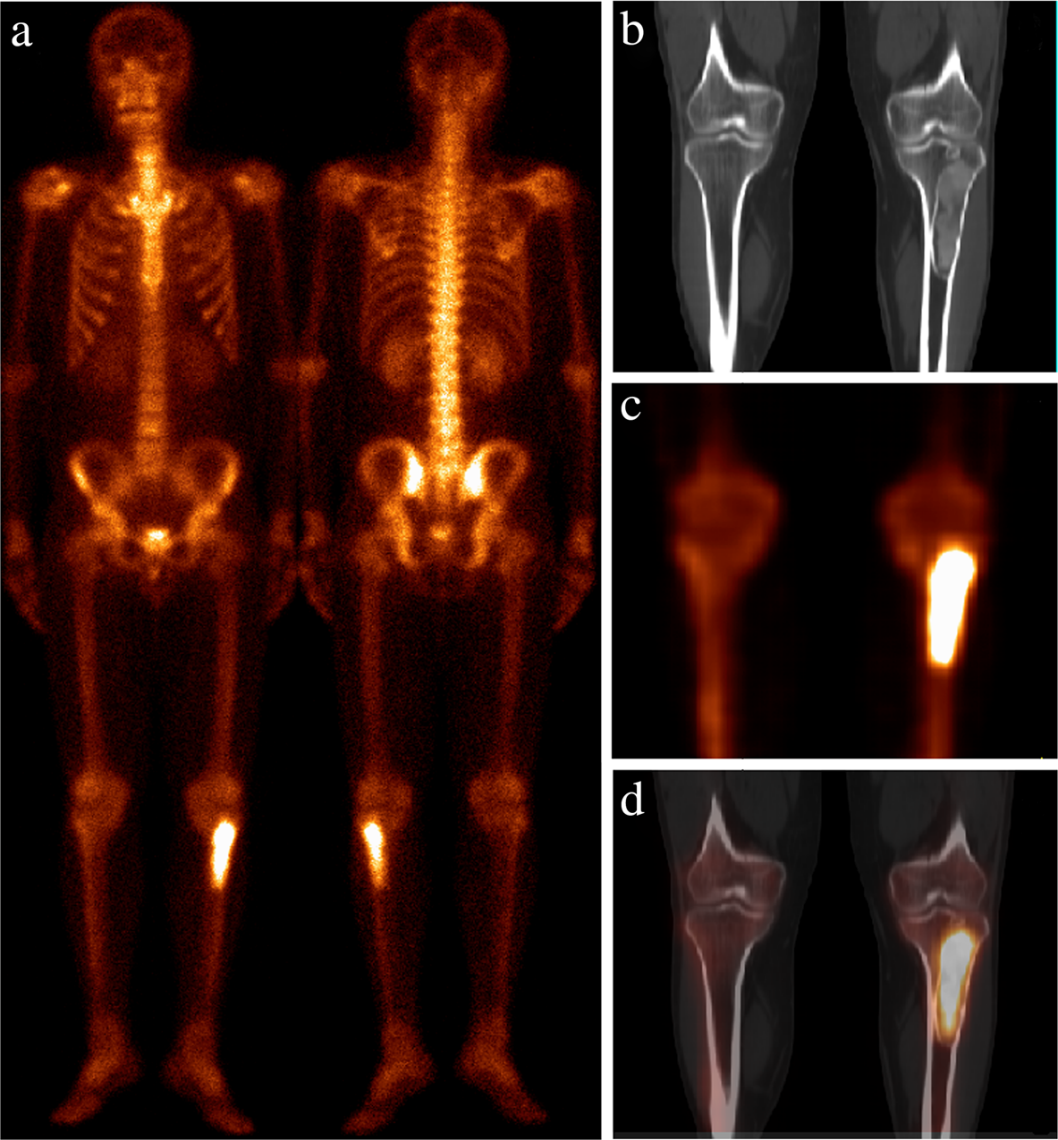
Patient 12 presented with NPC. On the WBS (a), there is an area of abnormal ^99m^Tc-MDP uptake seen in the left upper tibia, which may mimic bone metastasis. No additional area of abnormal ^99m^Tc-MDP uptake was identified on the remainder of the skeleton. Axial CT (b), SPECT (c), and hybrid SPECT/spiral CT imaging (d) depicted show increasing ^99m^Tc-MDP uptake corresponding to a sclerotic lesion with GGO localized in the left tibia. Based on the imaging finding which was suspicious for primary bone malignancy. A biopsy was subsequently performed and pathologic analysis confirmed the diagnosis of FD
